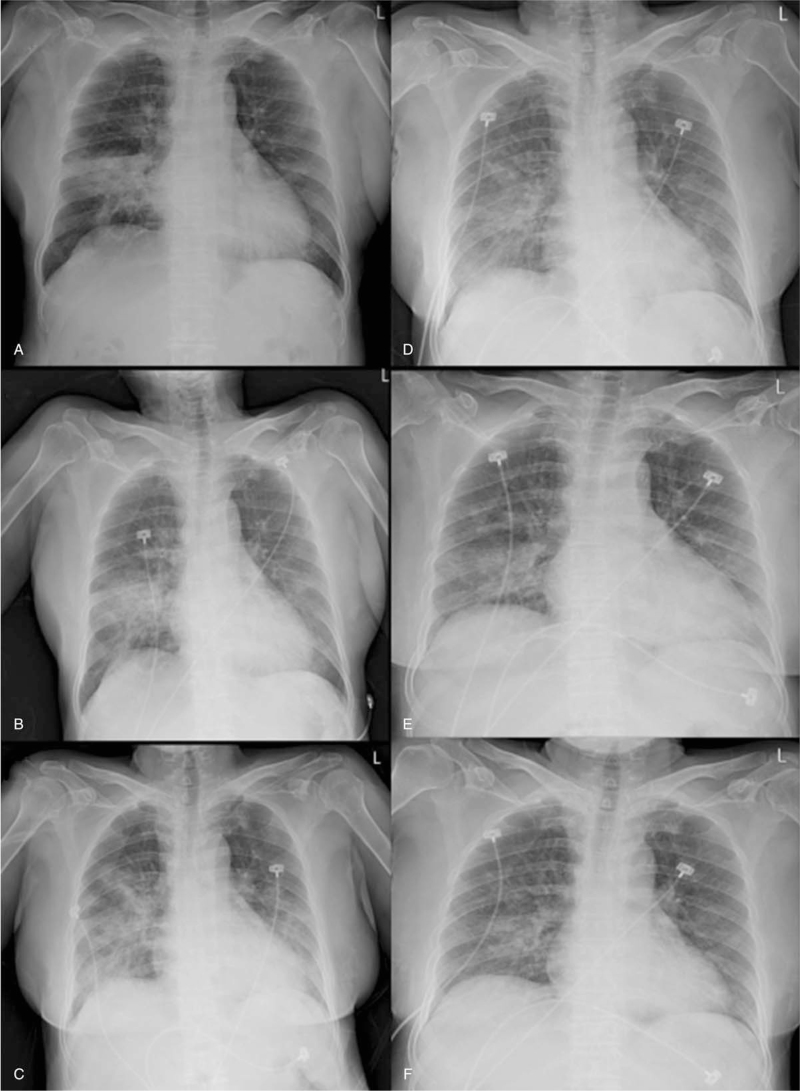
The results of serial chest X-rays in patients with COVID-19 pneumonia performed on illness day (A) 13 (hospital day 1), (B) 14, (C) 16, (D) 18, (E) 21, and (F) 23. COVID-19 = coronavirus 2019.
